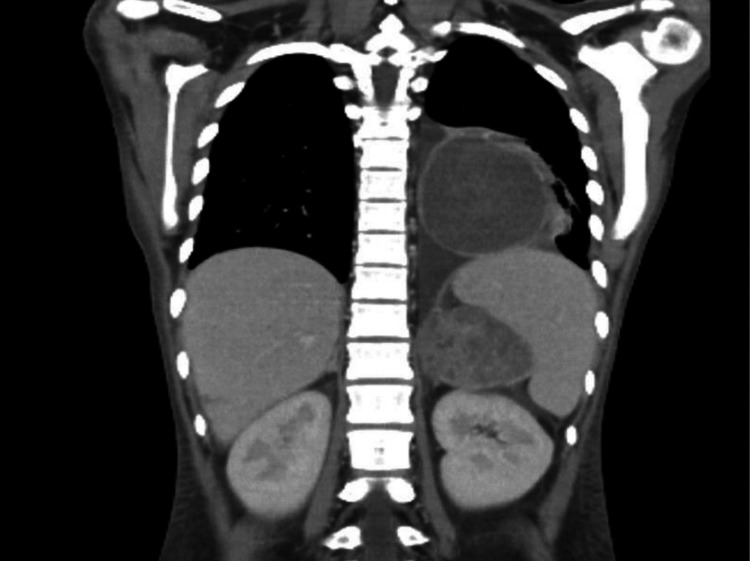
Coronal enhanced CT scan showing left adrenal and left lower lung lobe masses with unremarkable kidneys.
